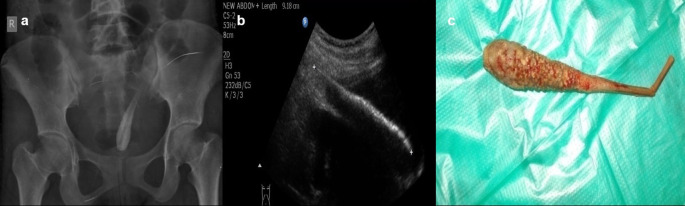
a–c: Radiograph Pelvis A-P view revealed long radio-opacity in the bladder with a radiolucent center - foreign body (a). USG revealed a linear hyperechoic foreign body that penetrated the anterior wall of the bladder (b). The removed foreign body was a long wooden stick with cotton wrapped around it (c).
